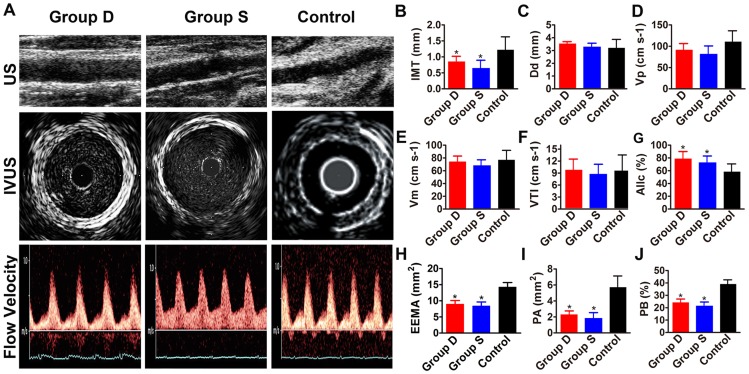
Ultrasonographic and intravascular ultrasound images and measurements in three groups of rabbits. Panel A shows two-dimensional ultrasonographic and intravascular ultrasound (IVUS) images and blood flow velocities of the abdominal aorta in three groups of rabbits. Panel B, C, D, E, F and G depict the measurements of IMT, Dd, Vp, Vm, VTI and AIIc in three groups of rabbits before pharmacological triggering. Panel H, I and J show the measurements of EEMA, PA and PB% in three groups of rabbits before pharmacological triggering. Group D: doxycycline-treated group; Group S: simvastatin-treated group; Control: control group.P*<0.05 vs. Control group.
